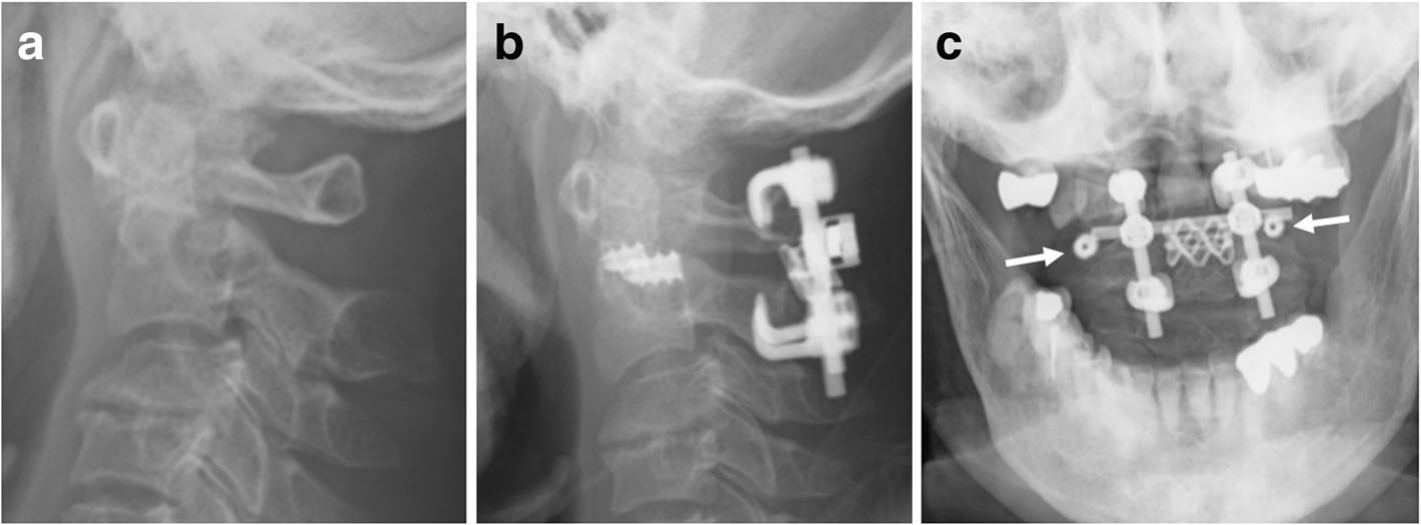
C1-C2 intra-articular screw fixation with a titanium mesh cage: an 80-year-old female with an odontoid fracture. a Lateral view on a plain radiograph before surgery. b Lateral view on a plain radiograph immediately after surgery. An intra-articular screw was inserted into the atlantoaxial joint. Hook-and-rod fixation was applied to the posterior region. c Anterior view on a plain radiograph immediately after surgery. The region between the posterior arch of the C1 vertebra and the lamina of the C2 vertebra was filled with a titanium cage and grafted bone. White arrow: the intra-articular screw in the atlantoaxial joint
